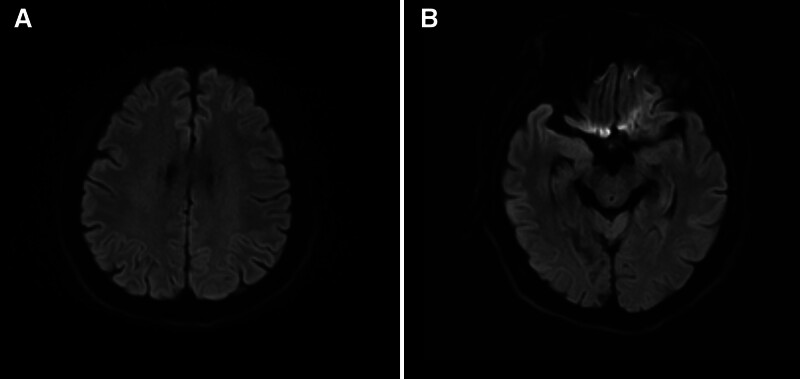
Baseline brain MRI obtained on the day of trauma. DWI sequences at the level of the cerebral ventricular body (A) and the suprasellar cistern (B) show no evidence of abnormal diffusion-restricted foci. DWI = diffusion-weighted imaging, MRI = magnetic resonance imaging.
